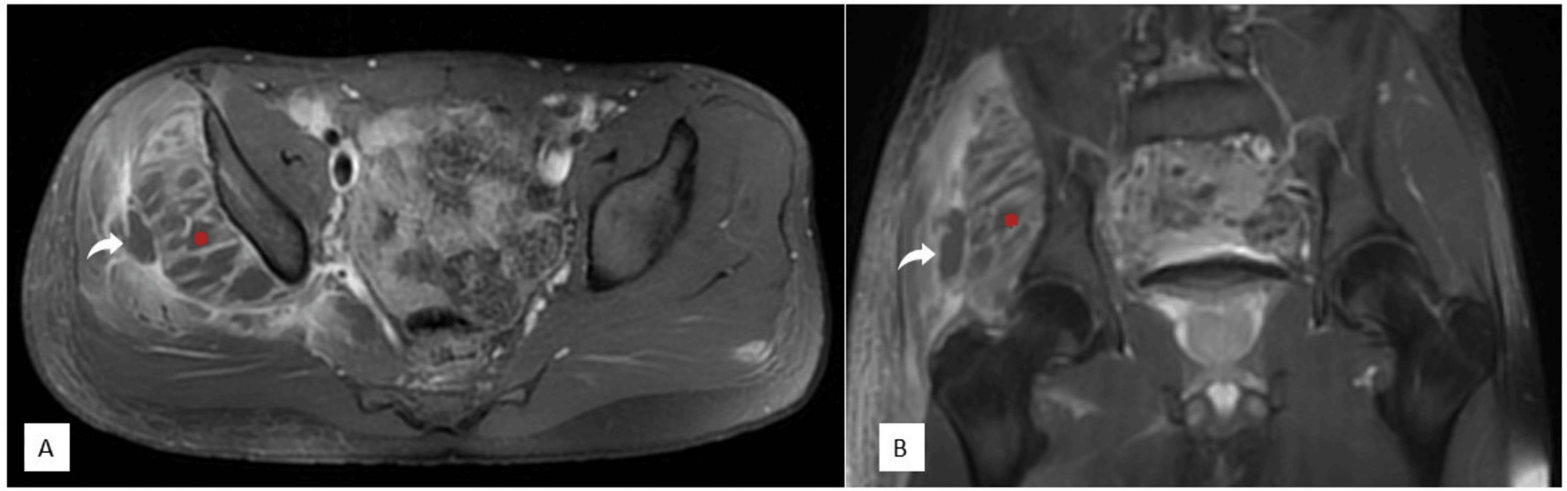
Post-contrast pelvis MRI showing (A) axial T1-weighted fat-suppressed and (B) coronal T1-weighted fat-suppressed images. The right gluteus minimus muscle (red asterisk) contains multiple small low-signal areas with peripheral enhancement, consistent with intramuscular fluid collections. A similar intermuscular collection is noted between the gluteus minimus and medius muscles (curved white arrow). Associated subcutaneous edema and enhancement are observed along the lateral aspect of the thigh. These findings are highly suggestive of an extensive inflammatory process with multiple intramuscular and intermuscular abscesses.
